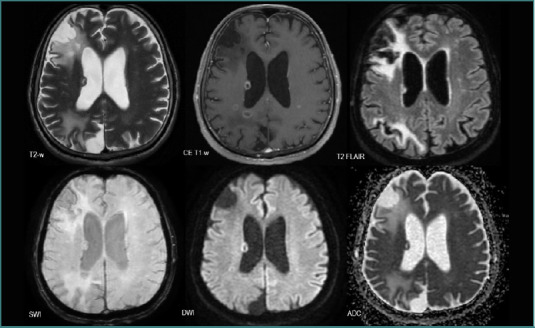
MRI brain acquisition of a patient with squamous cell carcinoma. Multiple lesions and a subependymal one on the external wall of the right lateral ventricle, with intense peripheric diffusion (high DWI and low ADC) and contrast intake with central necrosis (CE T1-w), without intralesional vascularization (SWI) and surrounding edema (T2-w, T2-w Flair). To note two porencephalic areas with peripheric gliosis suggesting ischemic stroke sequelae (right frontal and parietal).
